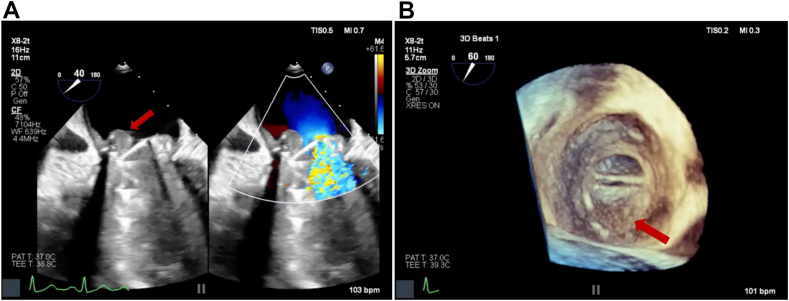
Two-dimensional TEE, mid-esophageal long-axis (40°) diastolic views (A) without (*left*) and with (*right*) color-flow Doppler, and (B) 3D TEE, short-axis (60°) volume-rendered reconstruction demonstrate a large mass (*arrow*, thrombus) on the mechanical MV restricting the opening of the posterior-facing mechanical disk (a shadowing artifact is also seen within the left ventricle beyond the prosthetic valve).
